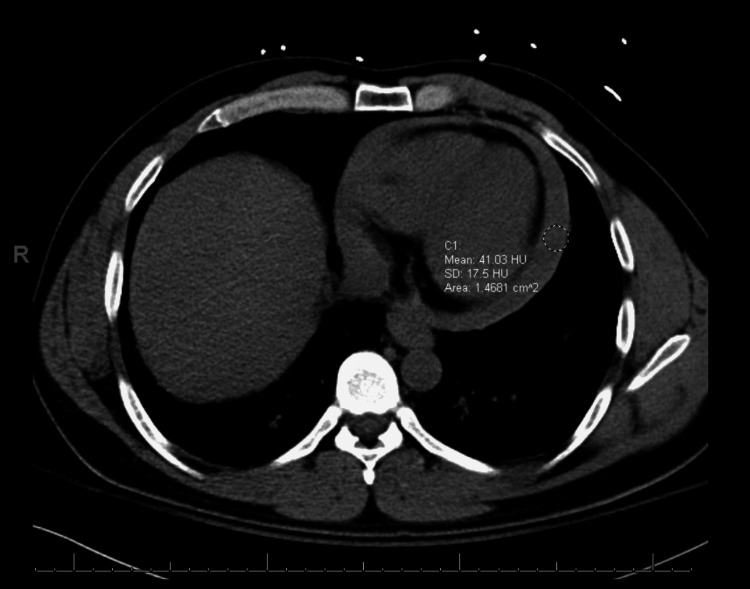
CT angiography of the chest demonstrating large hyperdense pericardial collection denoted as a circle.
